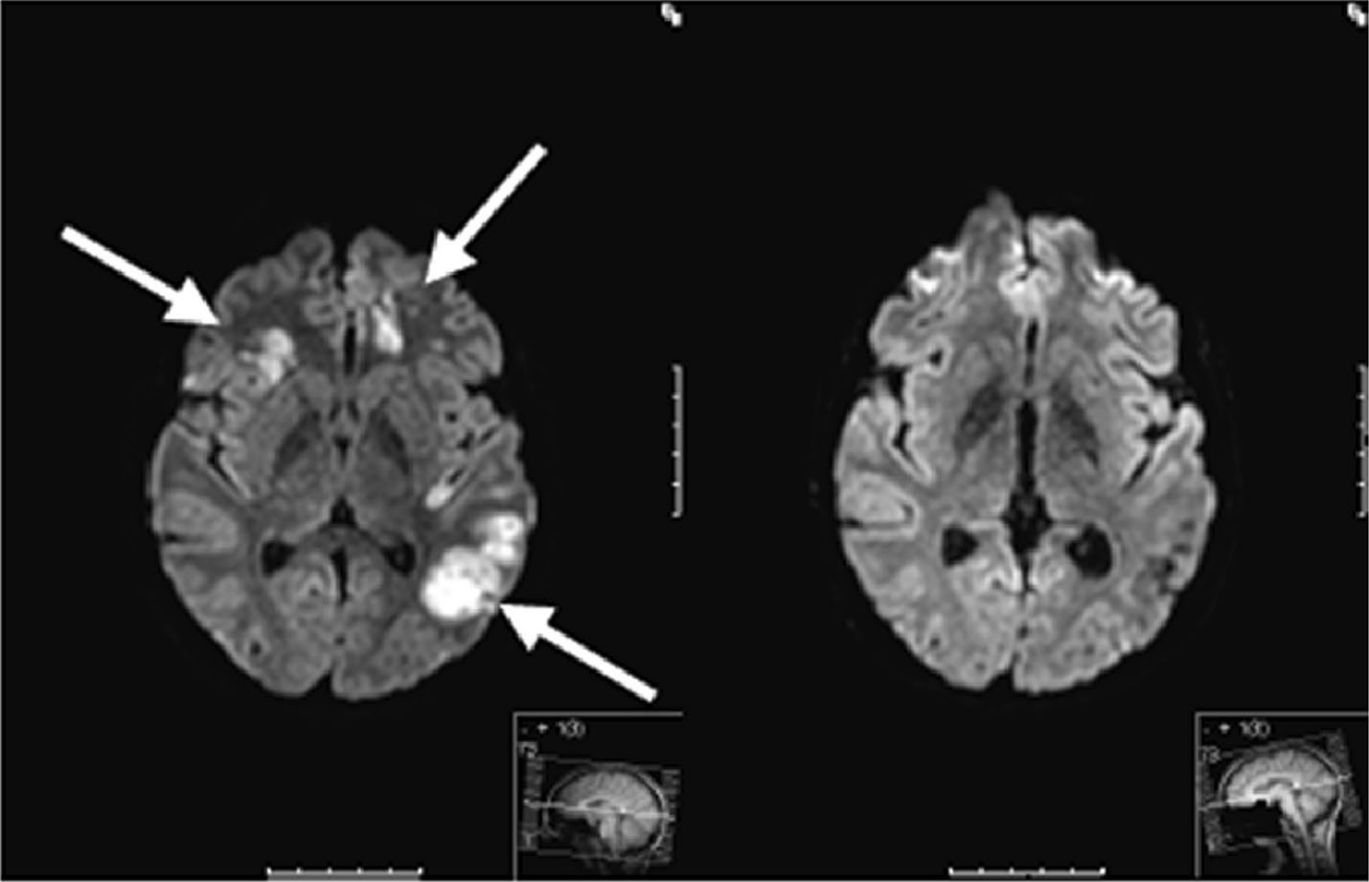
Axial T-2 sequences of a brain MRI scan of the 28-year-old patient. *Left* posttraumatic bihemispheric, mainly left-sided, ischemic lesions near the border zone, 3 days after trauma (indicated with *arrows*). *Right* 6 months follow-up MRI scan with good recovery.
